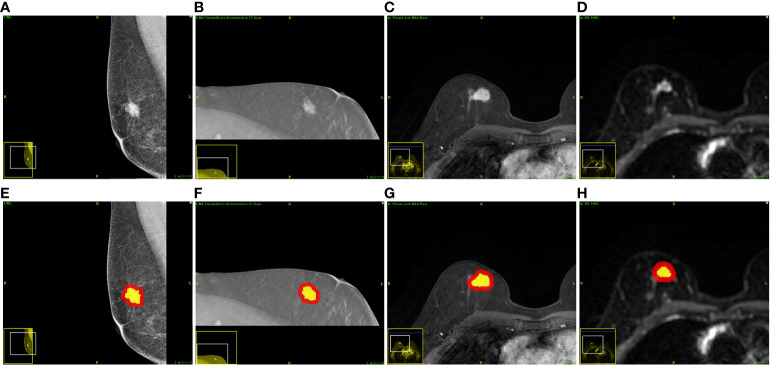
Examples of one patient who had invasive breast cancer and the segmented ROIs. From left to right: (A, E) the DM image, (B, F) the DBT image, (C, G) the DCE-MRI image, and (D, H) the DWI image. The yellow regions are the intratumoral ROIs. The red regions indicate the peritumoral ROIs.
